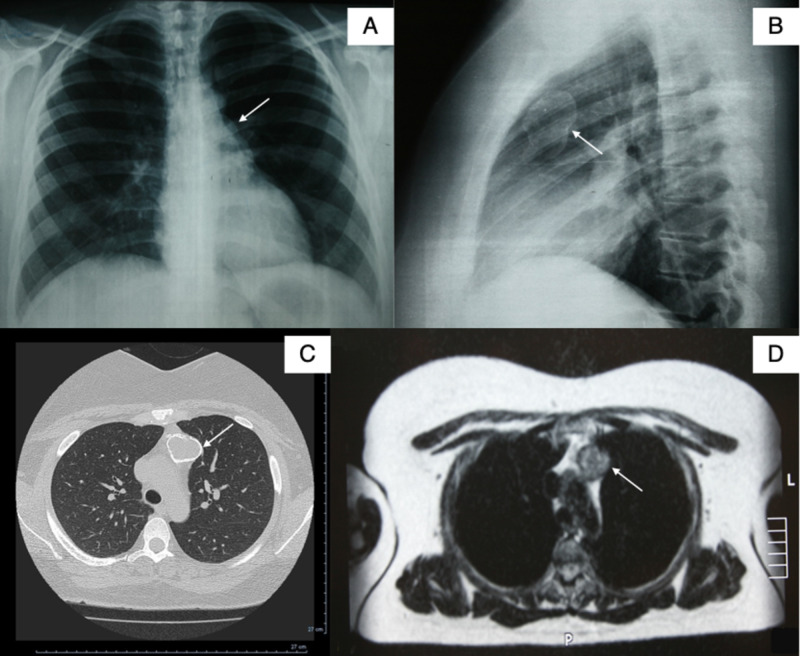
Chest X-ray, computed tomography, and magnetic resonance imaging A, B: Chest radiograph X-ray showing a calcified mass shadow; C: chest computed tomography showing an ellipsoid, well-defined, encapsulated cyst with low attenuation and rim calcification located in the anterior mediastinum; D: Magnetic resonance imaging showing a well-circumscribed cystic lesion with fluid content of low intensity on T1-weighted images and with no adhesion to the surrounding tissues
